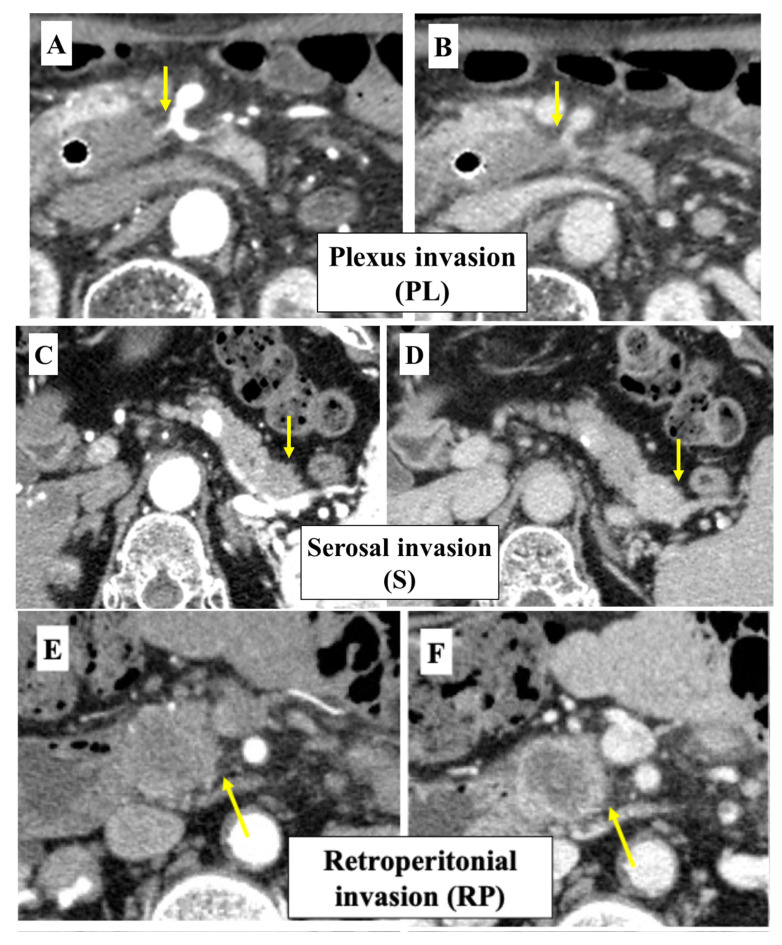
Representative CT findings of extrapancreatic invasion. (A,B) PL invasion (arterial and equilibrium phases). (C,D) S invasion (arterial and equilibrium phases). (E,F) RP invasion (arterial and equilibrium phases).
